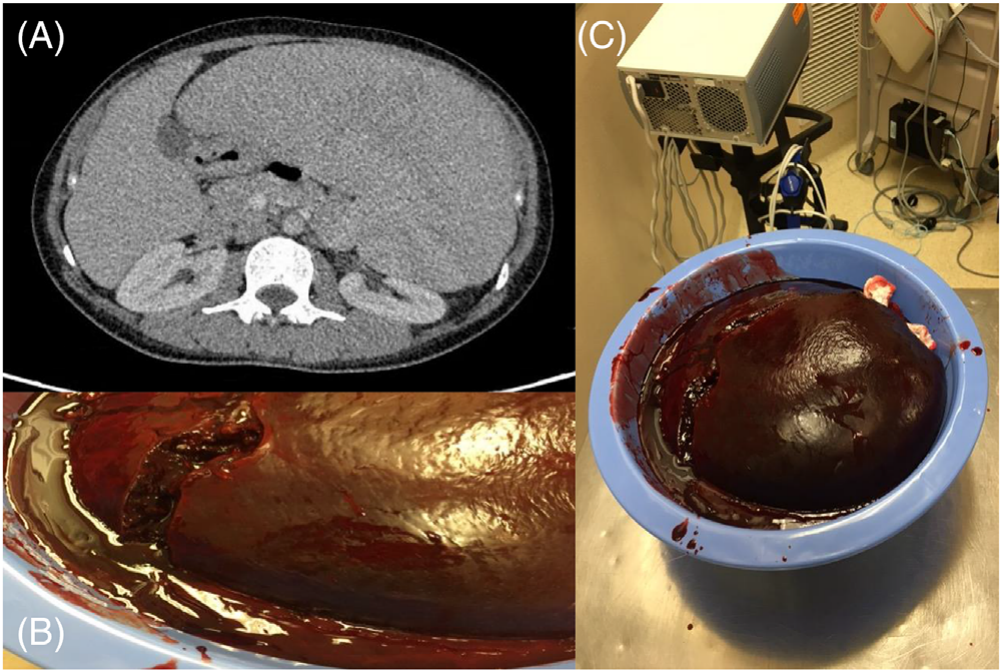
(A) CT abdomen‐image of massive splenomegaly. (B), (C) Massive spleen following resection
